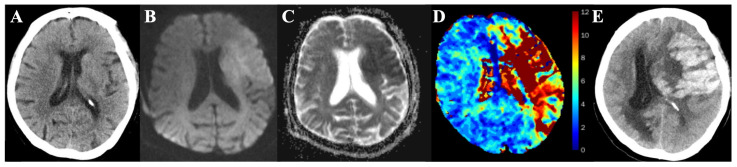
A representative AIS patient with HT in the follow-up CT. An 86-year-old female with right hemiparesis for 2 h. Intravenous thrombolysis therapy was performed after initial CT and MRI. No hemorrhage was found on the initial CT scan (A). Initial MR scan showed a patchy ischemic infarct area on the DWI (B) and ADC maps (C). Tmax map (D) showed the infarct area with delayed Tmax. HT was found after thrombolysis. Follow-up CT showed a high density of hemorrhage in the infarct area (E).
